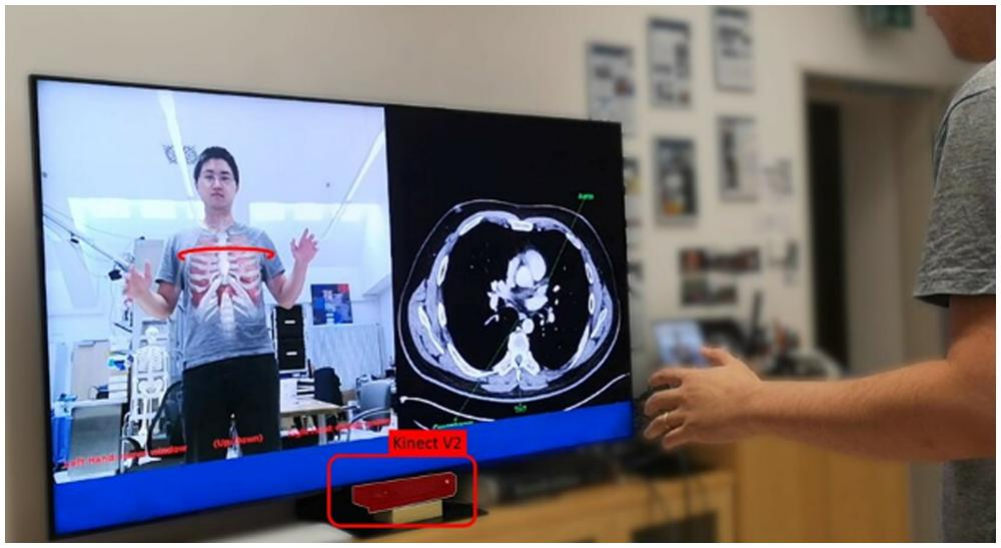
The so-called “Magic Mirror” shows the inner anatomy on the user’s “reflection” captured by an RGB-D camera. Alongside this projection, the mirror also shows transverse CT slices that can be selected by the user through hand gestures. Additionally, the pose of the user in front of the mirror distorts the virtual reflection of the user’s anatomy as it appears on the magic mirror.^11^
